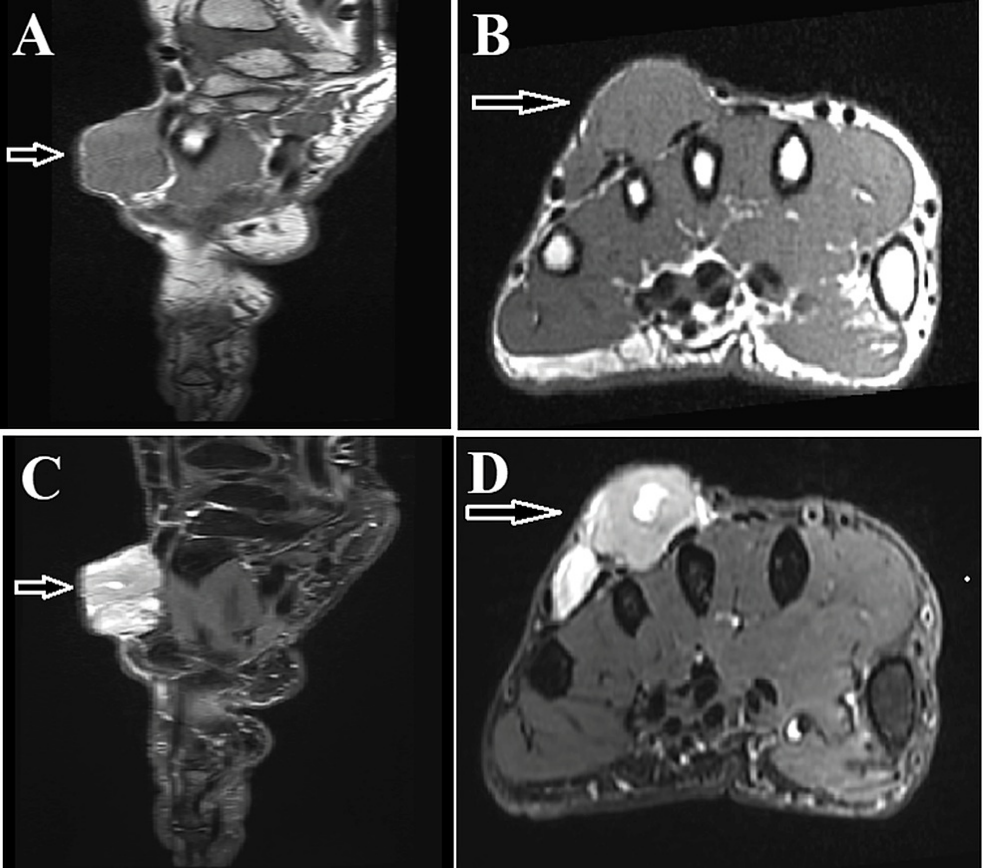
The magnetic resonance imaging (MRI) of the right hand demonstrates a lesion with low signal intensity in the T1-weighted image and a heterogeneous, high signal intensity in the T2-weighted image on the dorsum of the hand (indicated by white arrows). (A) Sagittal T1-weighted MRI image, (B) Axial T1-weighted MRI image, (C) Sagittal fat-suppressed T2-weighted image, (D) Axial fat-suppressed T2-weighted image.
